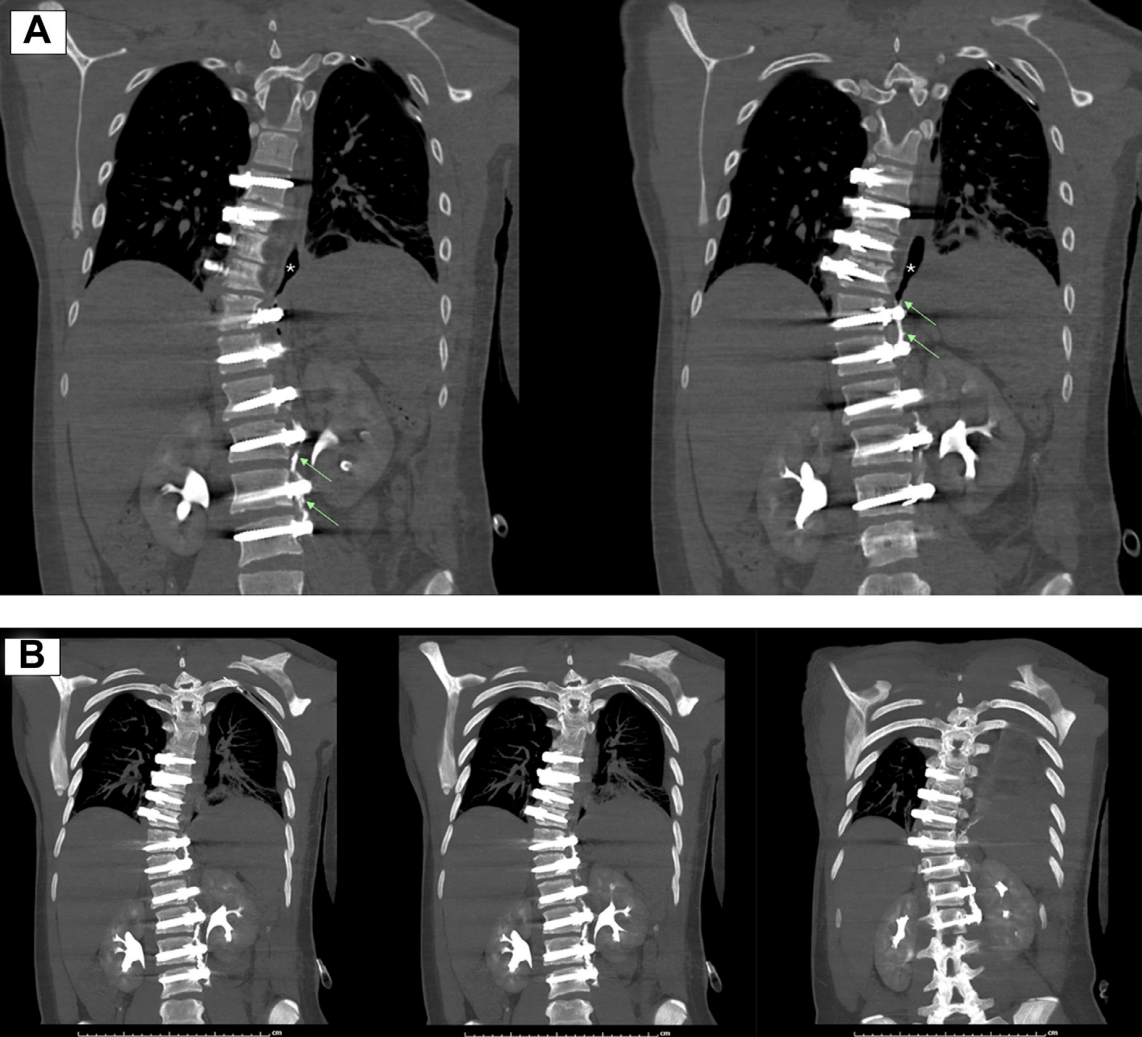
(A) CT chest/abdomen/pelvis without and with contrast, delayed phase imaging demonstrates a tract (arrow) of extraluminal contrast originating from the ureter traveling cranially into the pleural space (*). (B) Maximum intensity projections demonstrating nephropleural fistula tract.
